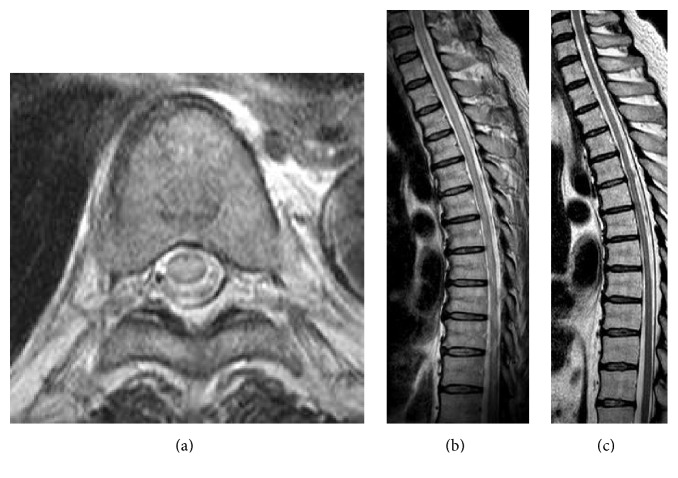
Characteristic spinal magnetic resonance images of Case 2. The thoracic spinal cord demonstrated longitudinal high-signal alterations with cord swelling prior to treatment ((a), (b)). After treatment, the spinal cord exhibited atrophic changes (c).
